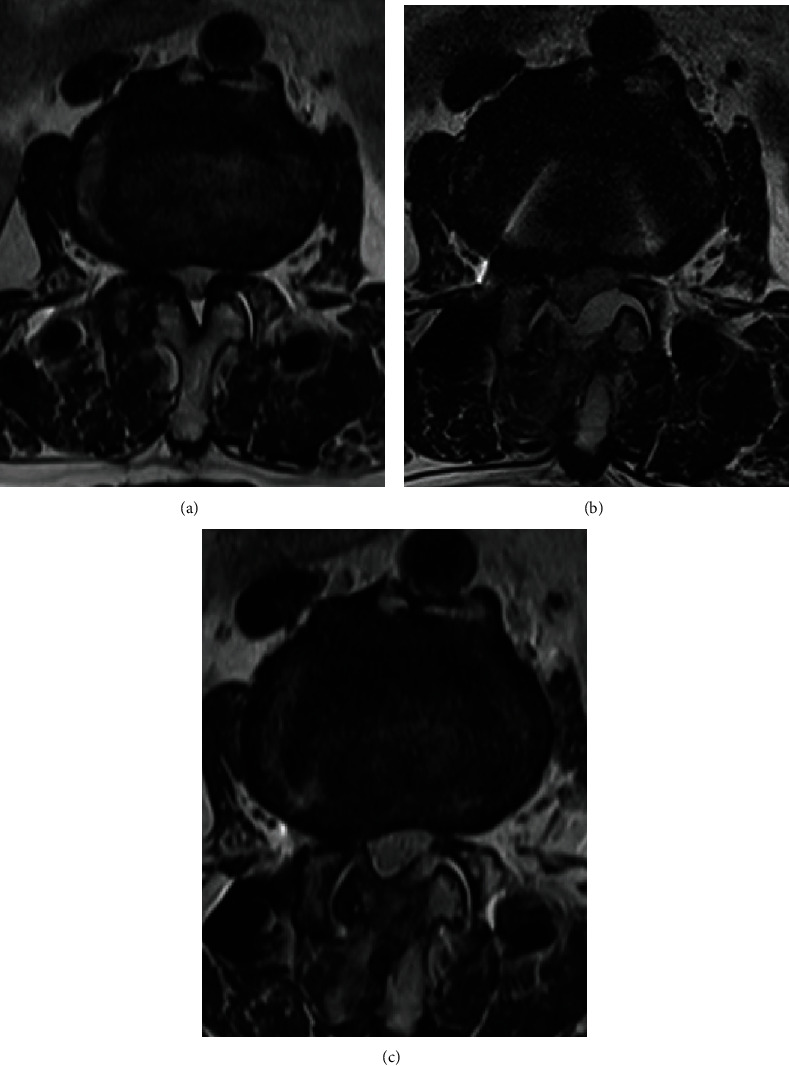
A patient who underwent BESS decompression for L2–3 spinal stenosis (a) Preoperative T2-weighted MRI showing bilateral recess stenosis. (b) Postoperative T2-weighted MRI showing grade II PSEH. (c) Follow-up MRI 6 months after surgery showing resorption of the PSEH with epidural fibrosis causing right lateral recess restenosis. (BESS: biportal endoscopic spine surgery, MRI: magnetic resonance image, PSEH: postoperative spinal epidural hematoma).
